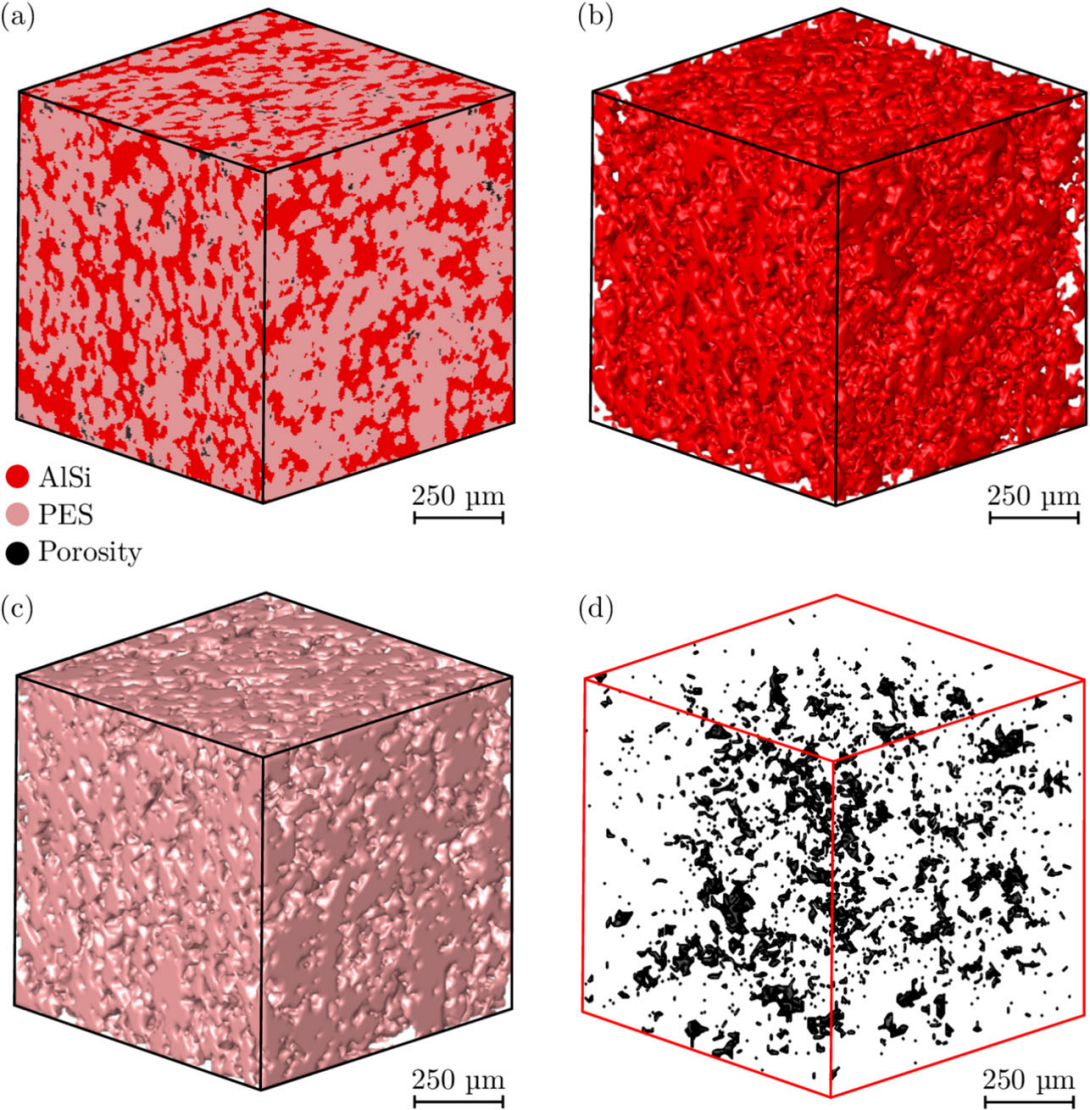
RVE extracted from X-ray CT scan of a compacted sample, with 1 mm edge length. (a) RVE, (b) AlSi phase, (c) PES phase, (d) Porosities
